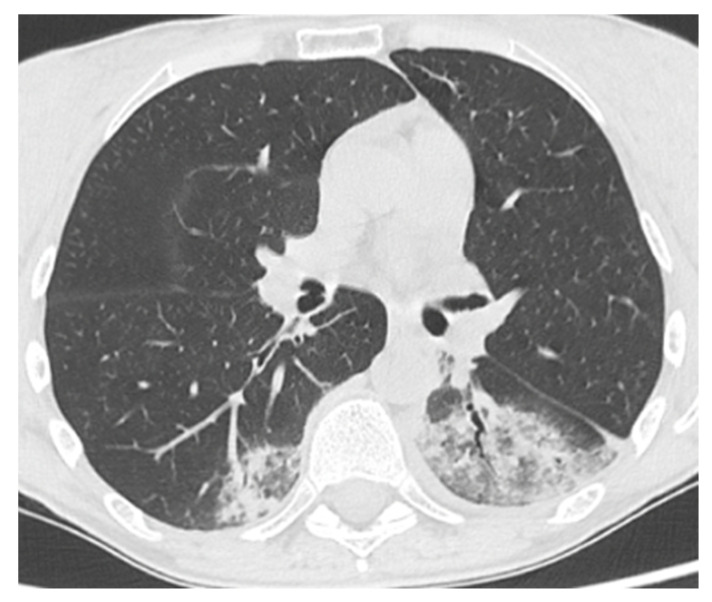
Patient 2: Chest CT scan at time of hospital admission for COVID-19 pneumonia, showing ground-glass opacities with inner foci of consolidation in both lower lobes.
